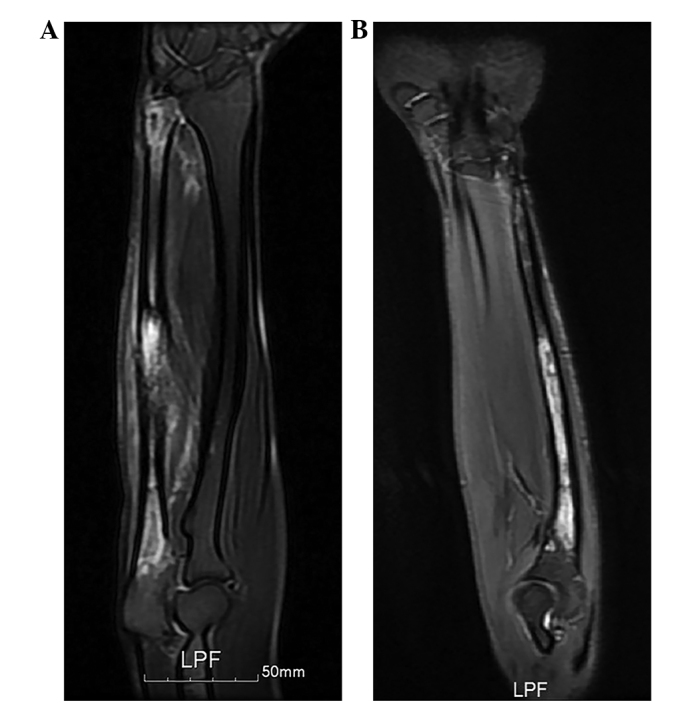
Magnetic resonance imaging findings (T2-weighted). (A) Prior to neoadjuvant chemotherapy. The intramedullary tumor involved nearly the full length of the ulna, with the exception of the proximal olecranon. The surrounding cortex was partially involved, and the soft-tissue components around the tumor appeared patchy and hyperintense. (B) Following neoadjuvant chemotherapy. The tumor regressed following two courses of intensive chemotherapy.
